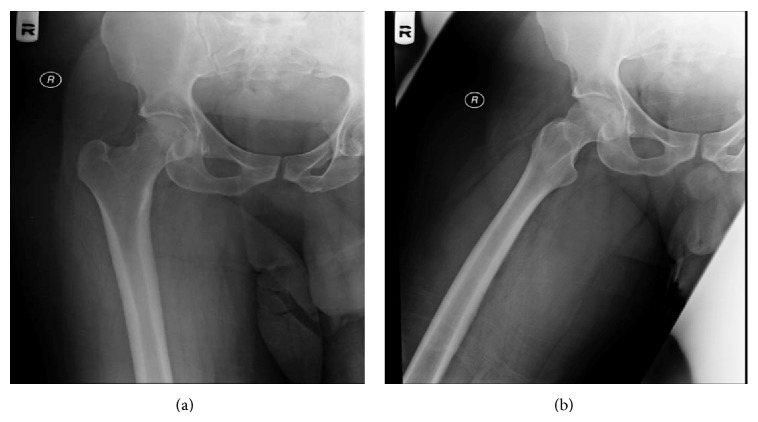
Right hip joint X-ray, (a) anteroposterior view and (b) abduction view.
